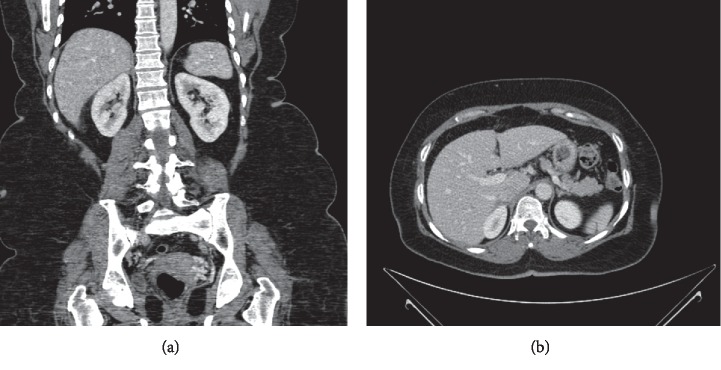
Postop CT adrenal: (a) coronal section and (b) axial section showed postresection of the right adrenal mass with no signs of residual or recurrent disease. The left adrenal gland is unremarkable.
